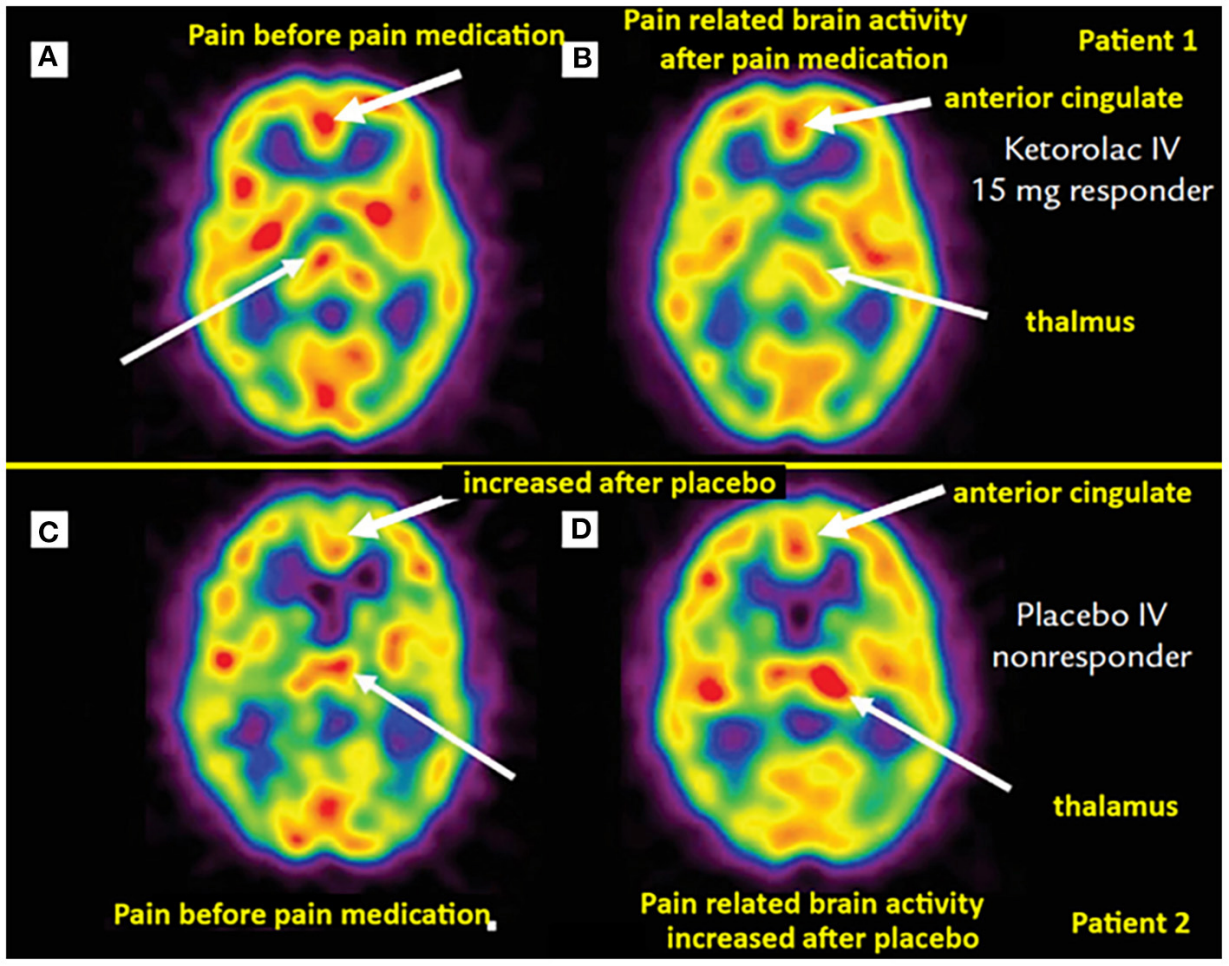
Brain perfusion SPECT of dental pain patients receiving analgesia (top row) vs. placebo (bottom row). (A) Asymmetric thalamic activity, more on the right (thin arrow). Post IV ketorolac, the post-interventional scan (B) of the same patient exhibits a slight “switch” in thalamic asymmetry, with mildly greater perfusion on the left (thin arrow). Noted also decreased perfusion in the anterior cingulate region with pain relief [thick arrows in (A) and (B)]. (C) Scan from another patient demonstrating mild asymmetric increased activity in the left thalamus (thin arrow). (D) Same patient with worsening pain after receiving IV placebo, the scan demonstrates more asymmetrically increased perfusion in the left thalamus (thin arrow). Not also increased perfusion in the anterior cingulate cortex compared to (C) (thick arrows). [Images by Newberg et al. (58), reproduced here with permission].
